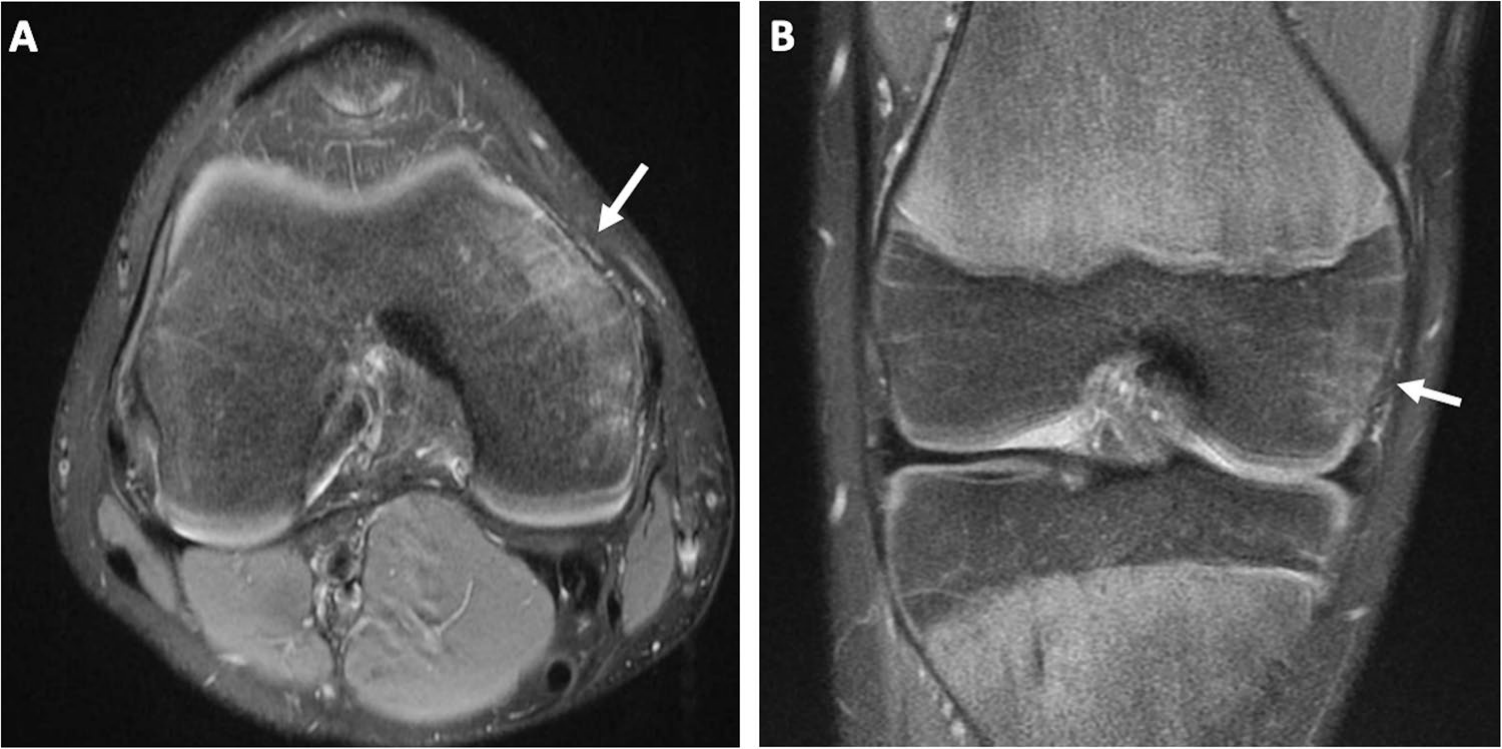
Magnetic resonance imaging of the right knee in a 13-year-old boy. Axial (a) and coronal (b) proton density fat-saturated images demonstrate marrow edema involving the anterior aspect of the medial femoral condyle (*arrows*)
